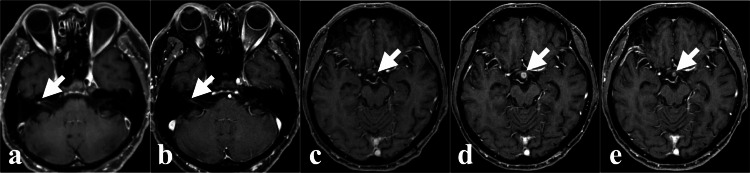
Time course of the right internal auditory canal and pituitary stalk lesions on enhanced axial MRI. (a) and (b) depict the right internal auditory canal lesion. (c), (d), and (e) depict the pituitary stalk lesion. (a) Enhanced lesion at CKR (indicated by the white arrow). (b) No lesion four months after CKR (indicated by the white arrow). (c) Enhanced pituitary stalk two months before CKR (indicated by the white arrow). (d) Increased enhancement of the lesion at CKR (indicated by the white arrow). (e) Shrinkage of the enhanced lesion four months after CKR (indicated by the white arrow). CKR: CyberKnife radiotherapy.
